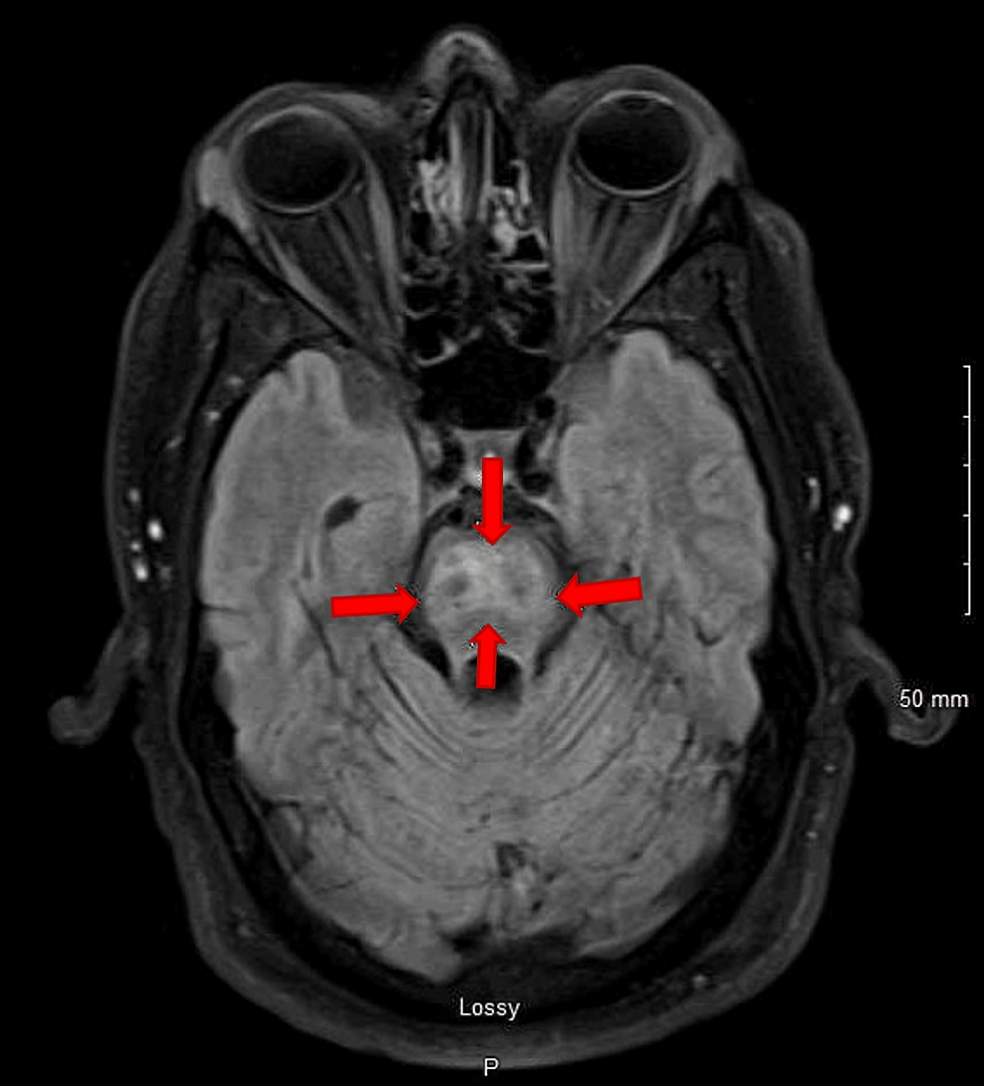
Arrows showing pontine hyperintensities on fluid-attenuated inversion recovery (FLAIR) sequence
